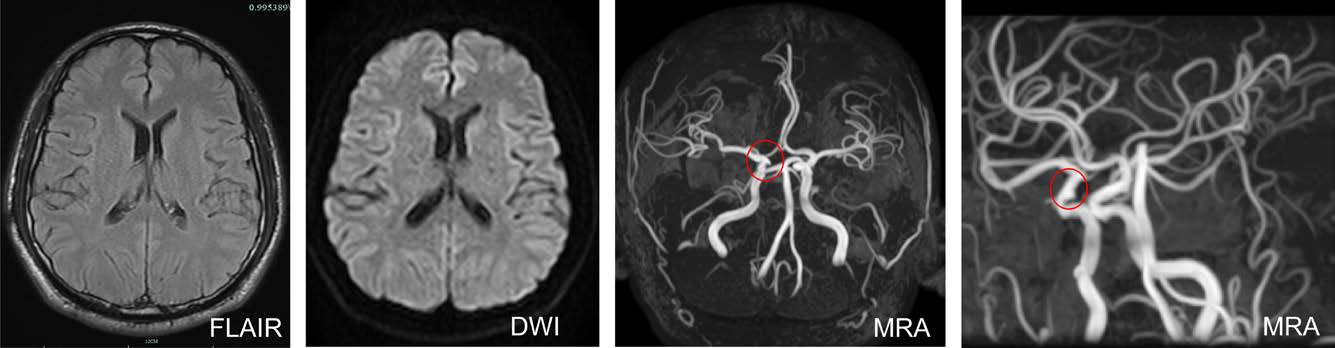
MRI Flair and DWI sequence is normal, and MRA showing an aneurysm (red circle) located at the ophthalmic segment of the right internal carotid artery. MRA = MR angiography, MRI = magnetic resonance imaging.
